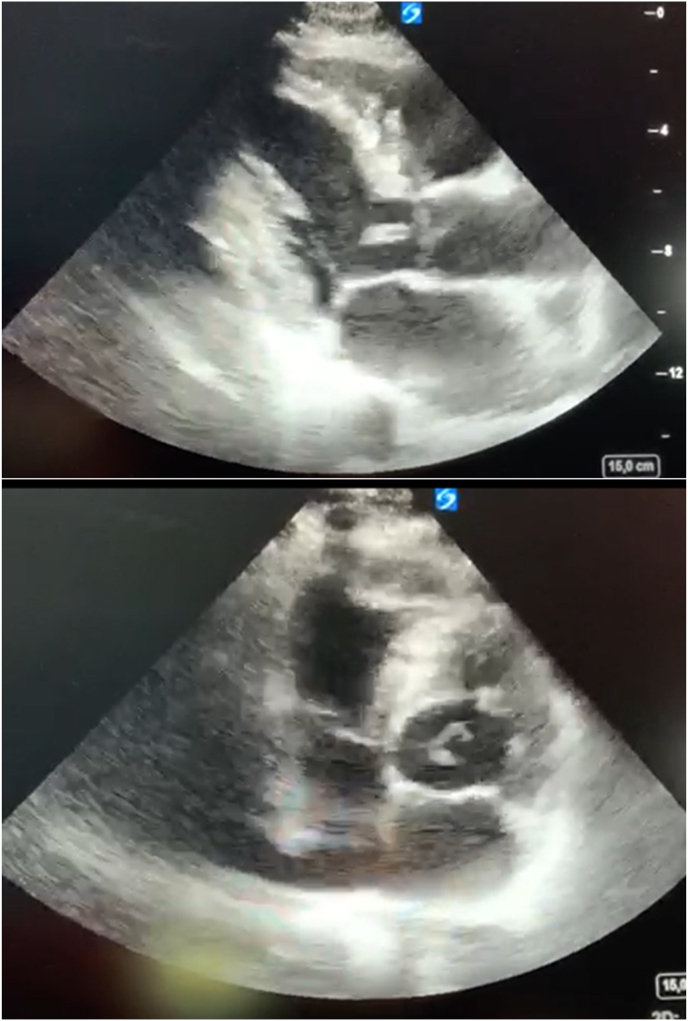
Echocardiography showed tri-commissural aortic valve with two vegetations appended respectively to the left coronary and non-coronary cusps measuring 3*34 mm and 4*41 mm in large diameter, with no significant leakage or stenosis No image of abscess or valvular destruction.
